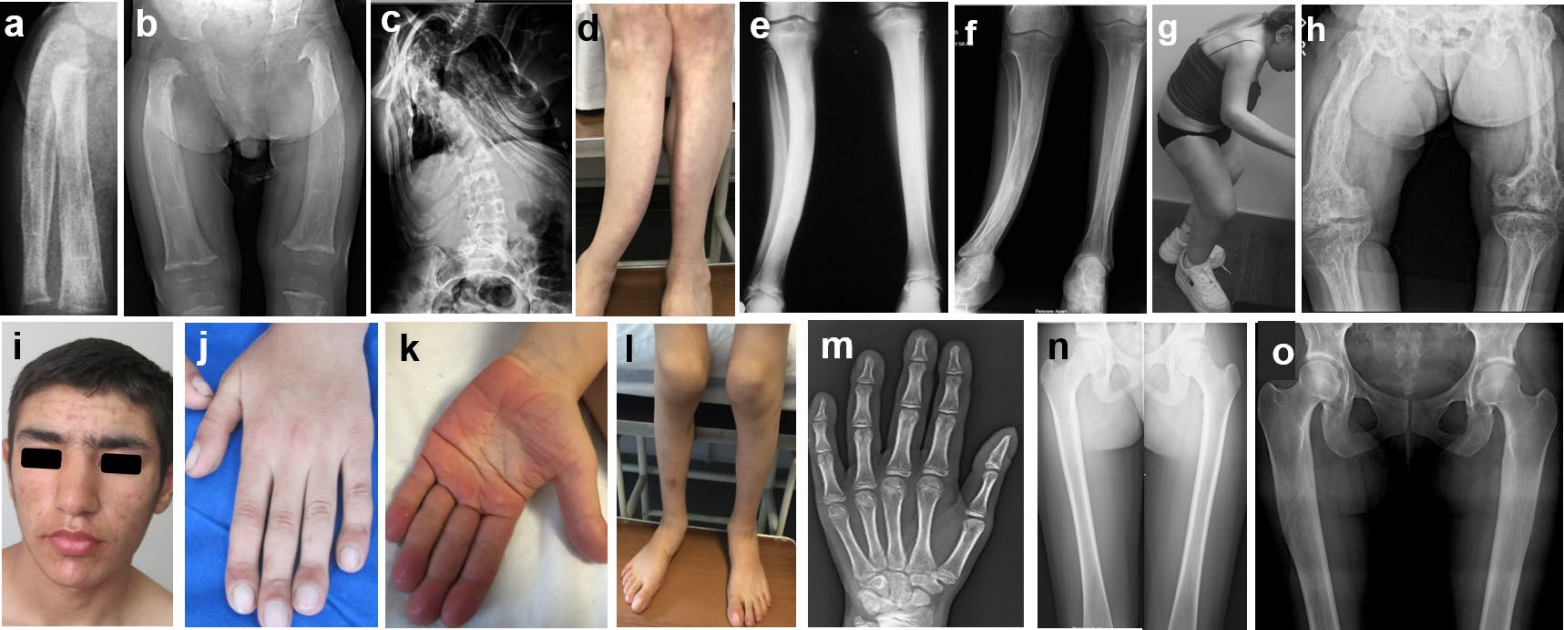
Photographs and radiographs of patients with Juvenile Paget’s disease-5 (a–h). Radiographs of P16 at 10 and 16 months of age, respectively (a, b) revealed osteopenia, multilayered, and irregular new bone formation at the diaphysis, and diaphyseal expansion. P14 showed severe scoliosis at the age of 23 years (c), anterior curvature of the tibia (d), and diffuse and patchy osteosclerosis of the long bones at the age of 13 and 23 years (e, f). P18 at the age of 30 years (g, h). Note the joint contractures with a forward bent posture with patchy osteosclerosis and coarse trabecular pattern. Photographs and radiographs of patients with hypertrophic osteoarthropathy type 1 (i–o). P24 at 17 years of age (i–o) showed coarse facial features (i), digital clubbing (j), enlargement and pachyderma of the hands (k), swelling of the knee (i–l). P22 at the age of 13 years (m, n), and P26 at the age of 24 years (o) showed acro-osteolysis (m) cortical thickening and periosteal hyperostosis (n, o)
